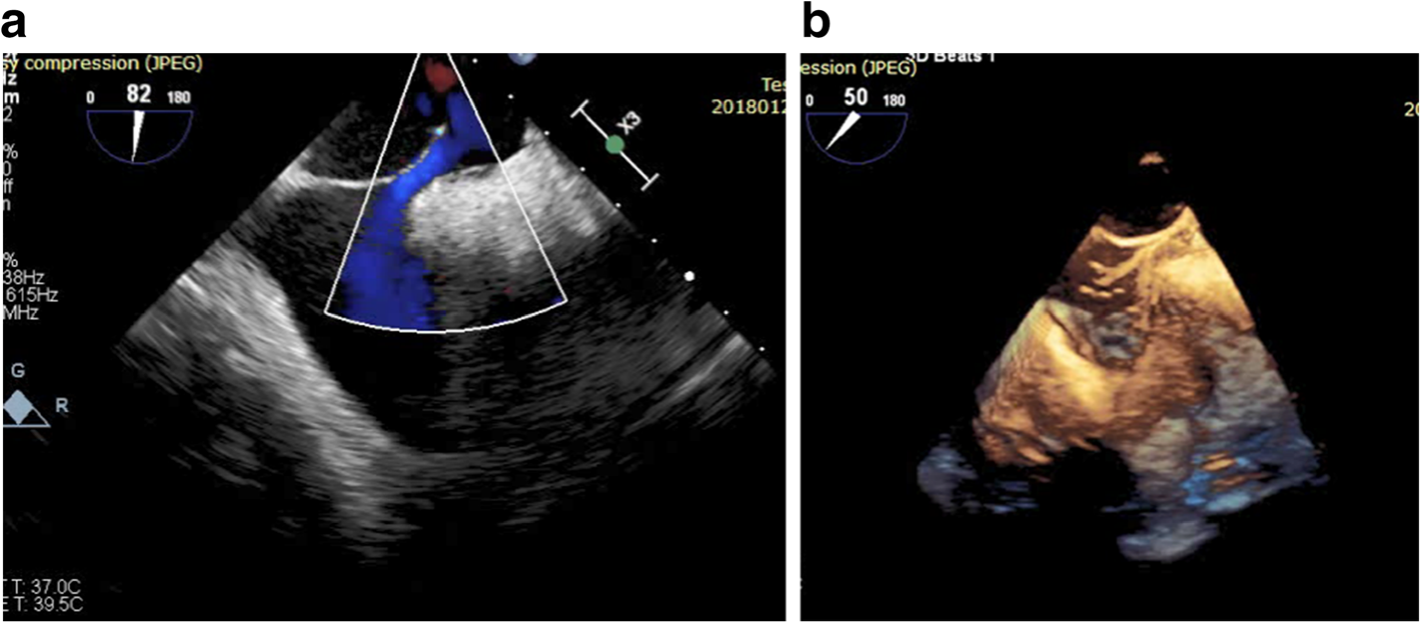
a 2D trans-esophageal echocardiography with color flow mode in bicaval view showing the wire across the patent foramen ovale (PFO). b Live 3D trans-esophageal echocardiography in bicaval view showing the wire across the patent foramen ovale (PFO)
